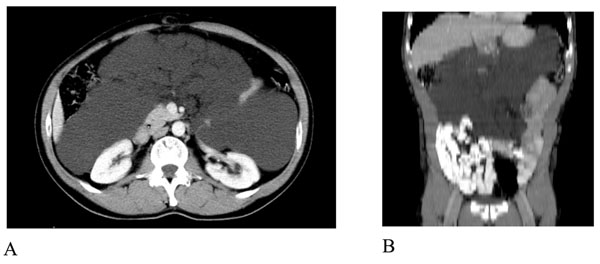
CT of the abdomen. Axial (a) and MPR coronal (b) CT images showed a huge mass composed by multiple confluent cystic lesions occupying the peritoneal spaces and displacing inferiorly the small bowel.
